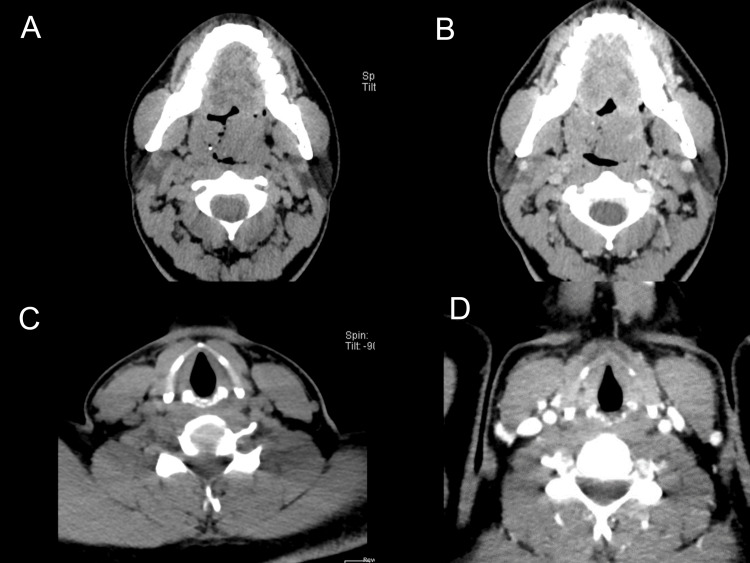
CT scan of the oropharynx (A and B) and the neck (C and D) (A) CT scan of the oropharynx. (B) CT scan of the oropharynx with contrast. (C) CT scan of the neck. (D) CT scan of the neck with contrast. CT: computed tomography
